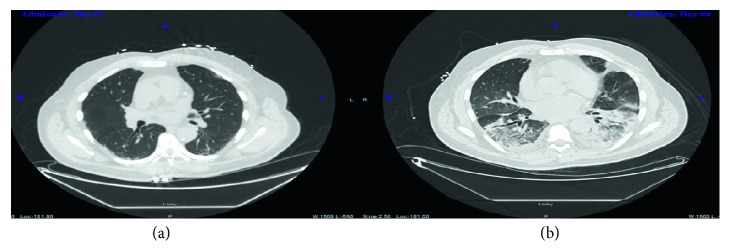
Contrast-enhanced chest computed tomography. (a) No filling defect in the main or lobar branches of the pulmonary arteries. Evidence of mild subsegmental atelectasis in the lung bases is seen. There are no airspace infiltrates, focal consolidation, or pleural effusion. (b) New development of bilateral basilar infiltrates and consolidations without pleural effusion.
